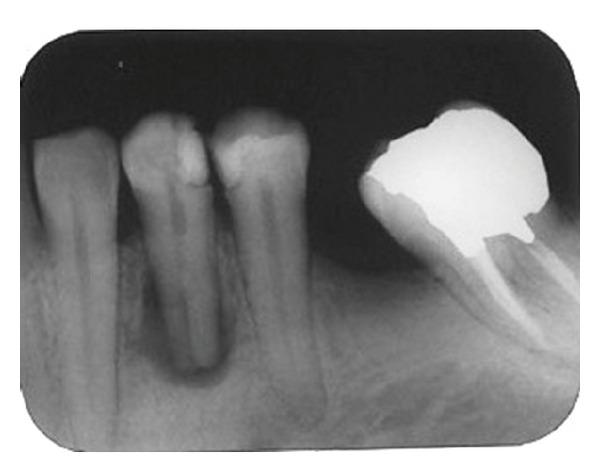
Periapical radiograph of mandibular left first premolar revealing complex root canal system.
